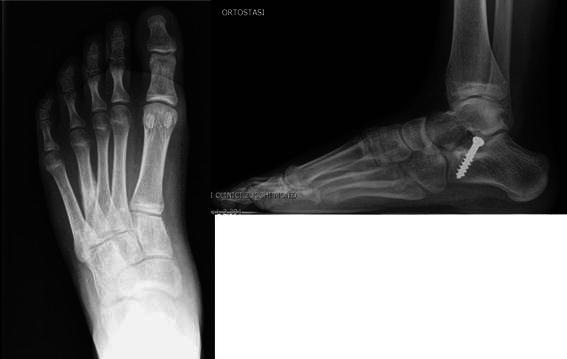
Stress fracture of the fourth metatarsal bone in a patient who underwent subtalar extra-articular screw arthroereisis (SESA). The patient mentioned pain along the lateral border of the midfoot which an X-ray 3 months after surgery confirmed to be due to a healed stress fracture
